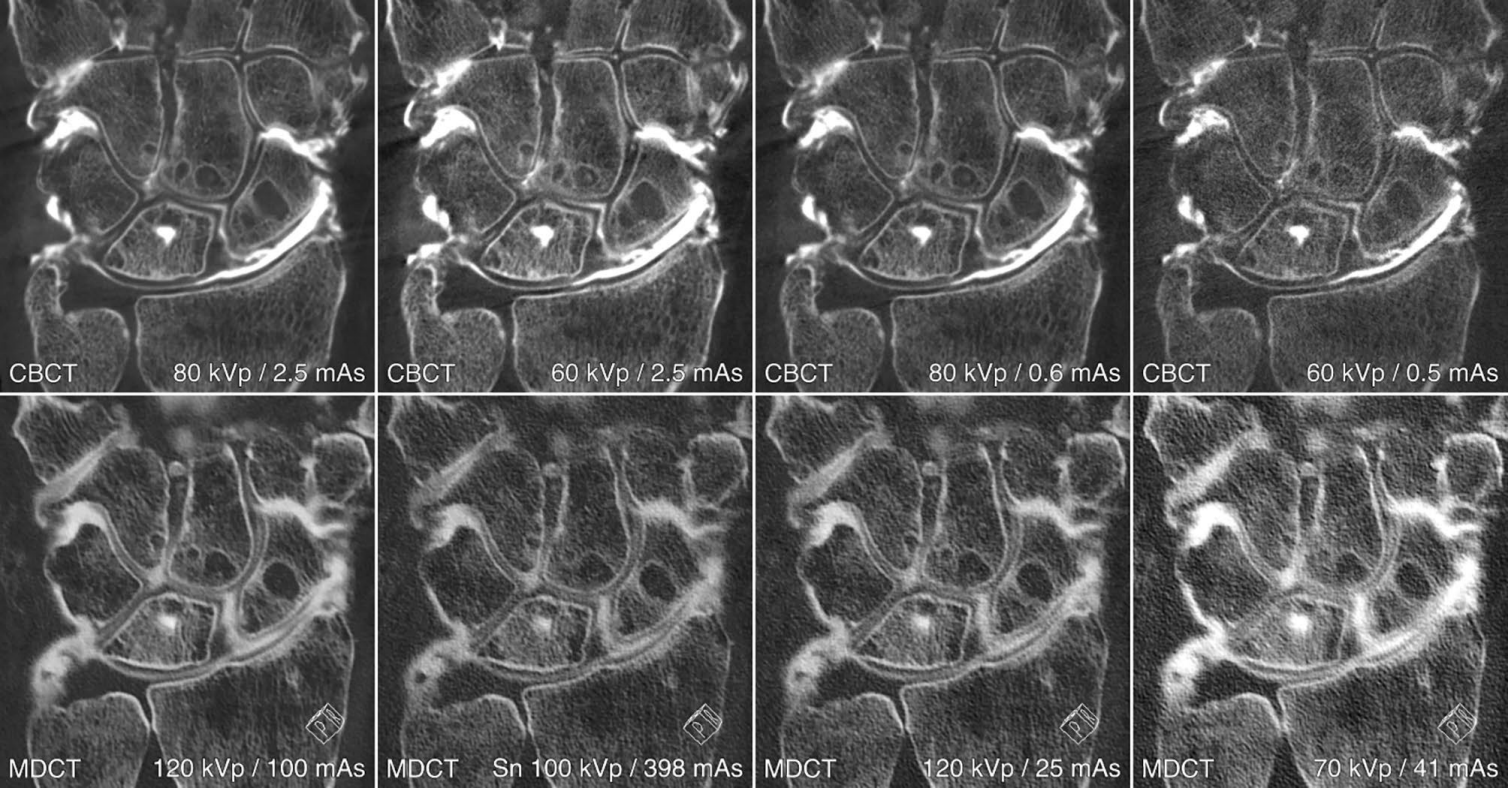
Coronal view of various scan protocols (in descending order of radiation dose) depicts a scapholunate ligament tear. Visualization of the tear’s location in the scaphoid portion of the ligament is superior in cone-beam CT scan (CBCT; upper row). Despite being acquired 30 minutes later, the blurriness of multidetector CT images (MDCT; lower row) partially offsets the advantage of increased articular distension for cartilage assessment, for example in the radiocarpal compartment.
